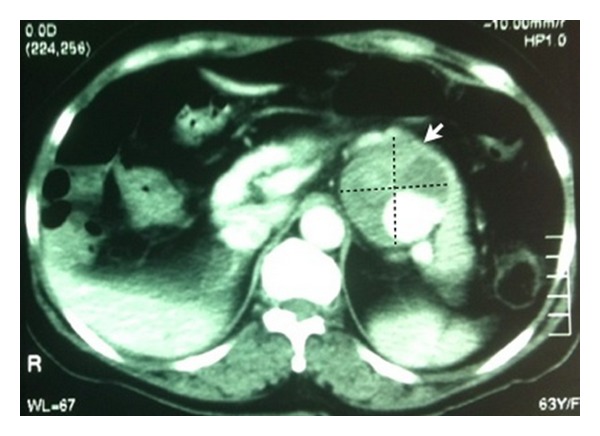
Axial section of CT of the abdomen with intravenous contrast. The arrow indicates the underlying lesion to the pancreas (splenic artery aneurysm).
